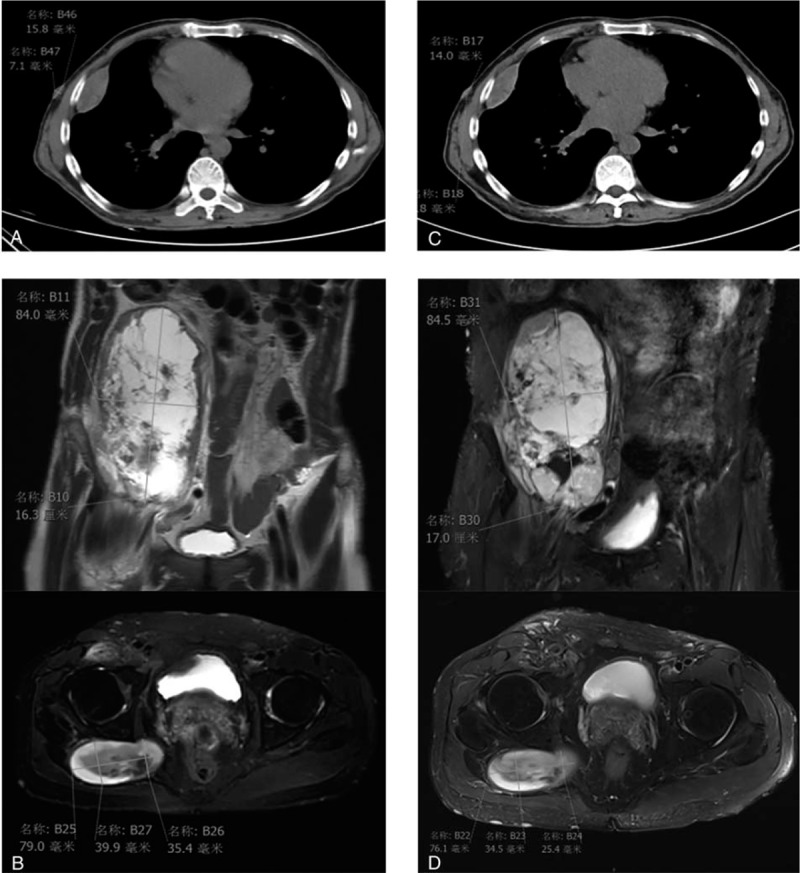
Before crizotinib treatment, CT scans (A), and abdomen MRI (B). After crizotinib treatment for 50 days, CT scans (C), and abdomen MRI (D). CT= computed tomography , MRI = magnetic resonance imaging.
